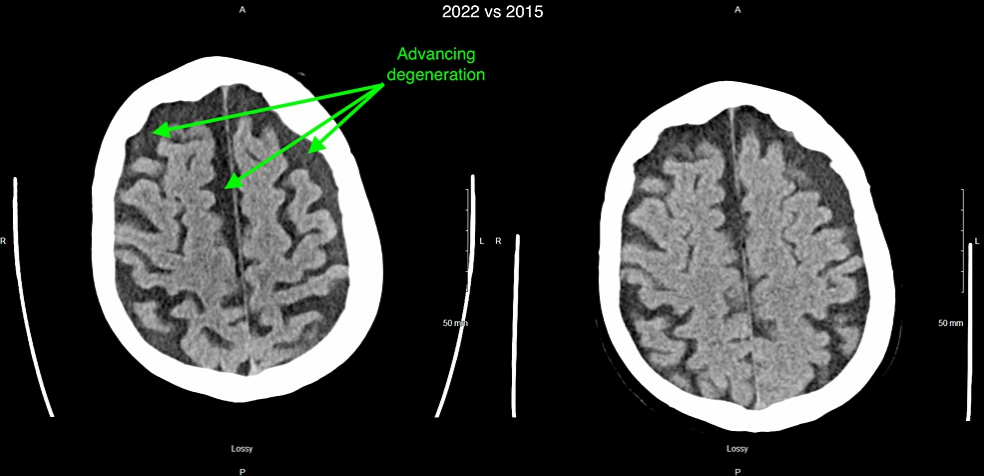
Computed tomography (CT) without contrast of the head 2022 vs 2015 (4) 2022 scan is on the left while 2015 scan is on the right
